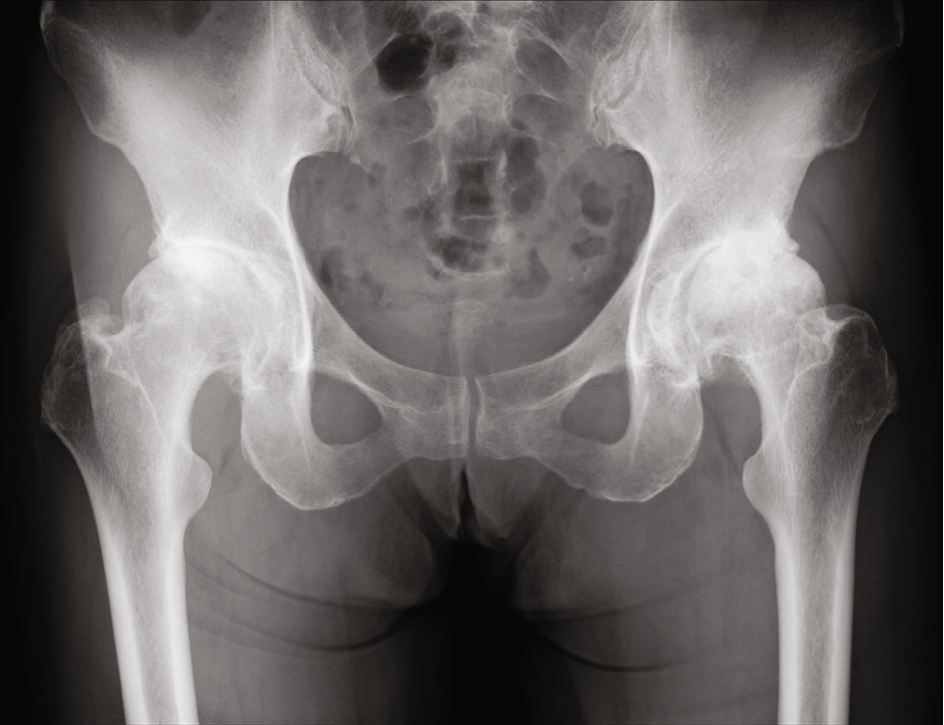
The plain radiograph of initial visit to our hospital at 7 years before surgery. Osteoarthritic changes in both hips without acetabular dysplasia were identified.
